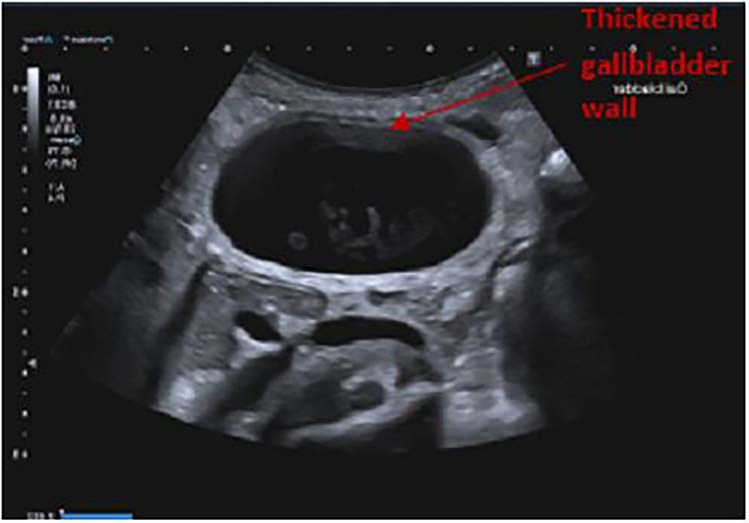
Ultrasound abdomen showing a thickened gallbladder wall.
